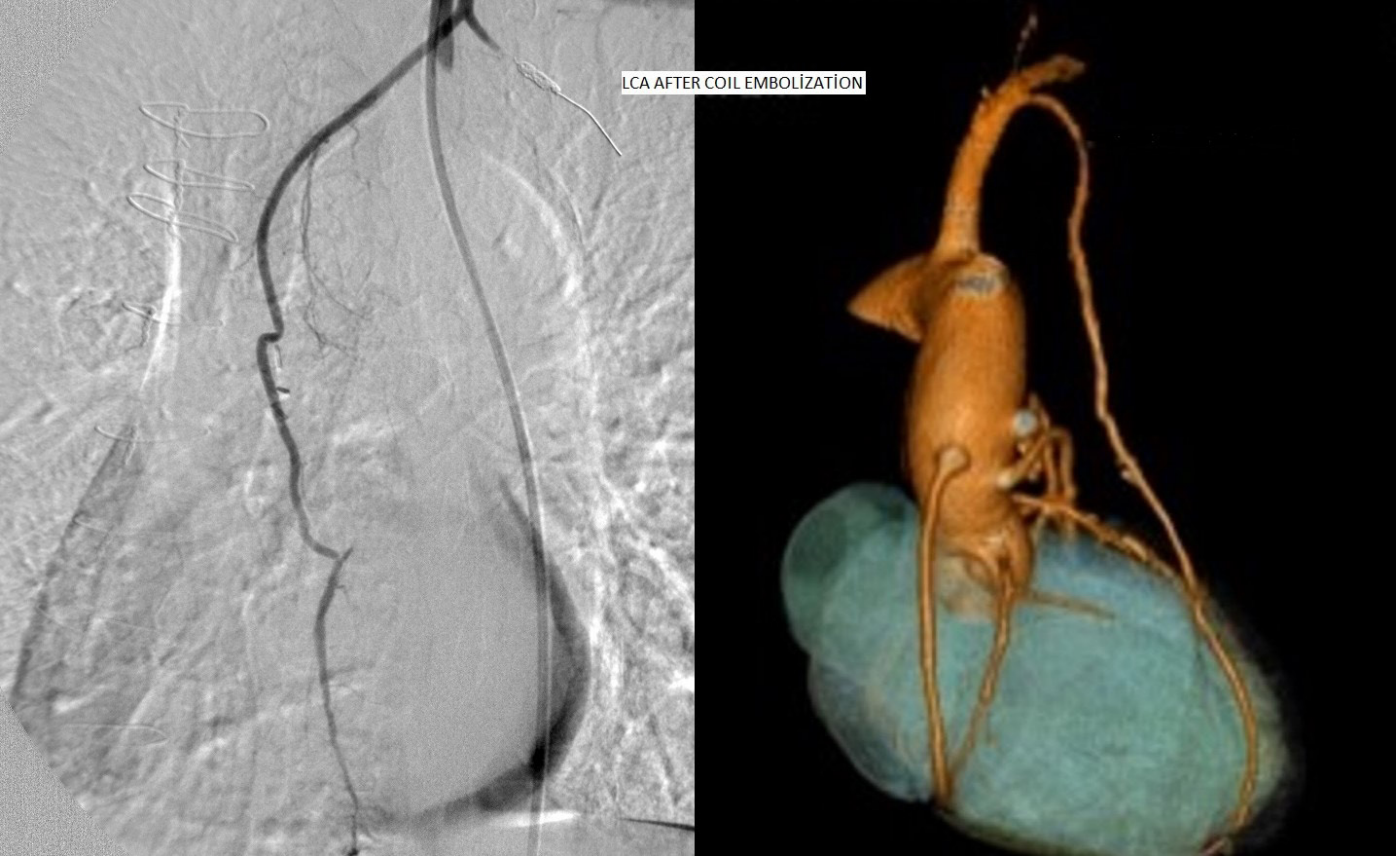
Cineangiographic and computed tomography angiographic view of the LITA
